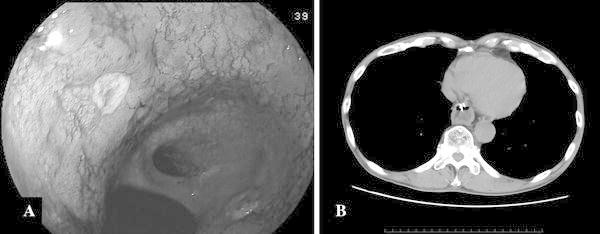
Gastrointestinal fiberscopy and CT images of the gastric tube cancer. a The gastric tube cancer was located on the anterior wall of the antrum. b The gastric tube cancer was located behind the* left* atrium on computed tomography, and a clip was placed for radiotherapy
